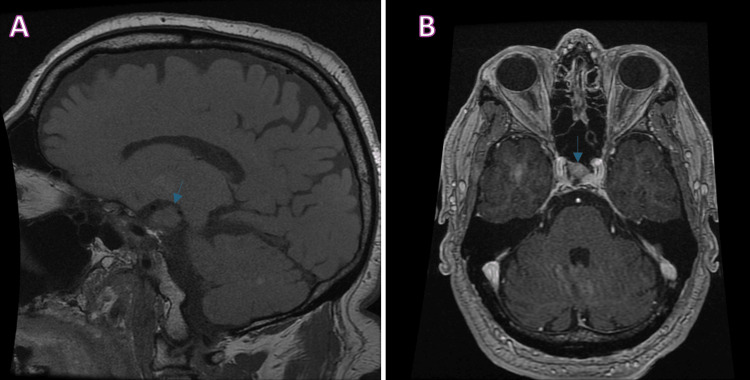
MRI brain showing pituitary macroadenoma A) T1 pre-contrast sagittal scan showing an ill-marginated lesion (blue arrow) of the sella measuring 0.8 x 1.3 cm; B) T1 post-contrast axial scan showing an ill-marginated hypoenhancing lesion (blue arrow) along the right side of the sella measuring 0.8 x 0.8 cm
